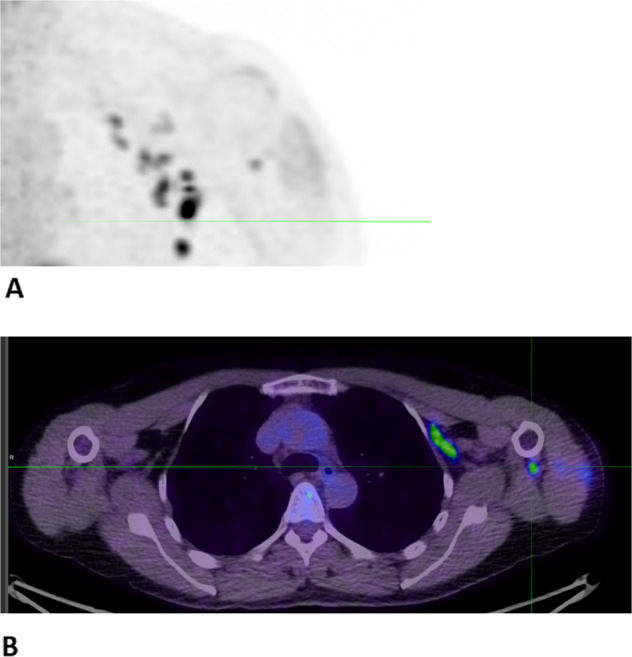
Immune activation imaged by FDG-PET. FDG-PET/CT images of a patient FDG-avid left axillary lymphadenopathy after COVID-19 vaccination, reflecting glucose metabolism in immune cells. In the maximum intensity projection image (panel A), lymph nodes appear as black spots and in the transverse PET/CT image (panel B) as light green regions.
